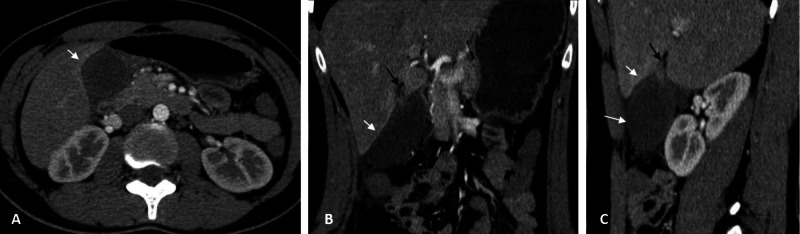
Gallbladder CT A review of the gallbladder CT in the arterial phase, showing axial (A), coronal (B), and sagittal (C) views with a beak sign at the cystic pedicle of the gallbladder (black arrow), slightly floating gallbladder (long white arrow) without horizontal laying of the gallbladder. Note the enhanced rim of the liver capsule (short white arrow) separating from the non-enhanced gallbladder wall is a thin layer of fluid. Abbreviation: CT, computed tomography
